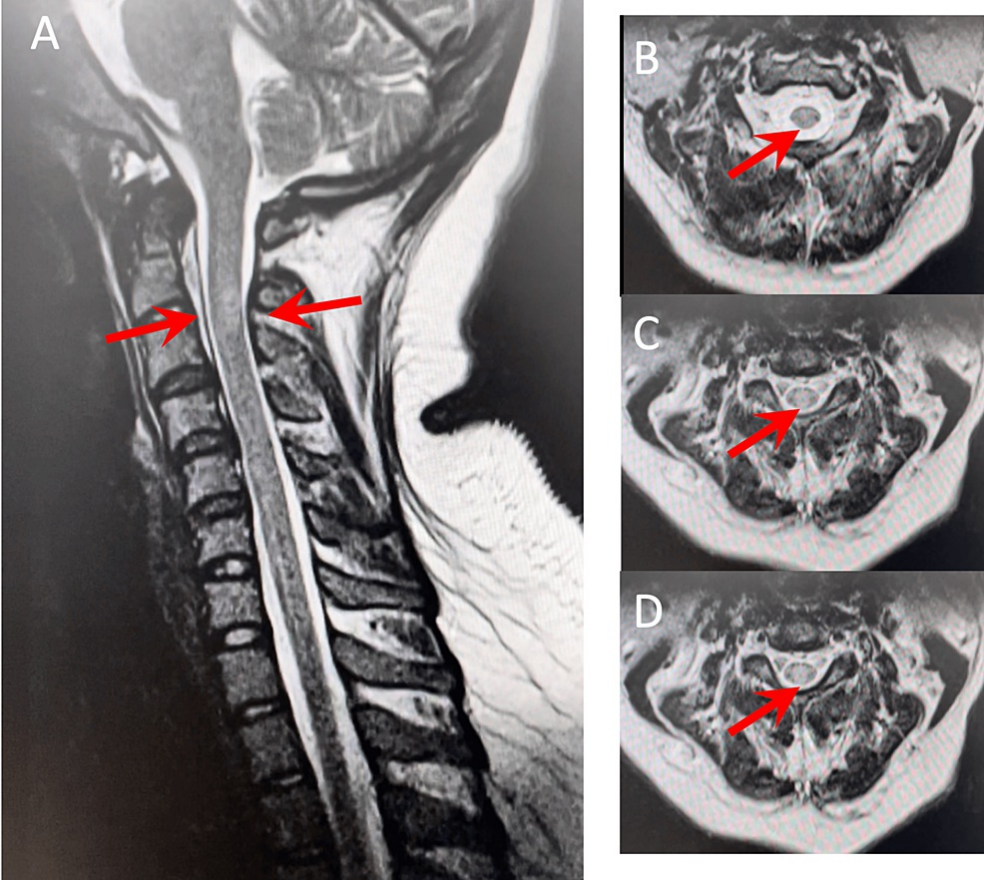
T2 weighted cervical MRI showing focal ovoid signal abnormality involving the dorsal aspect of the C2-3 level of the upper cervical spinal cord A: T2 weighted cervicothoracic MRI sagittal cut B: T2 weighted cervical MRI transverse cut at upper C2 C: T2 weighted cervical MRI transverse cut at lower C2 D: T2 weighted cervical MRI transverse cut at upper C3
